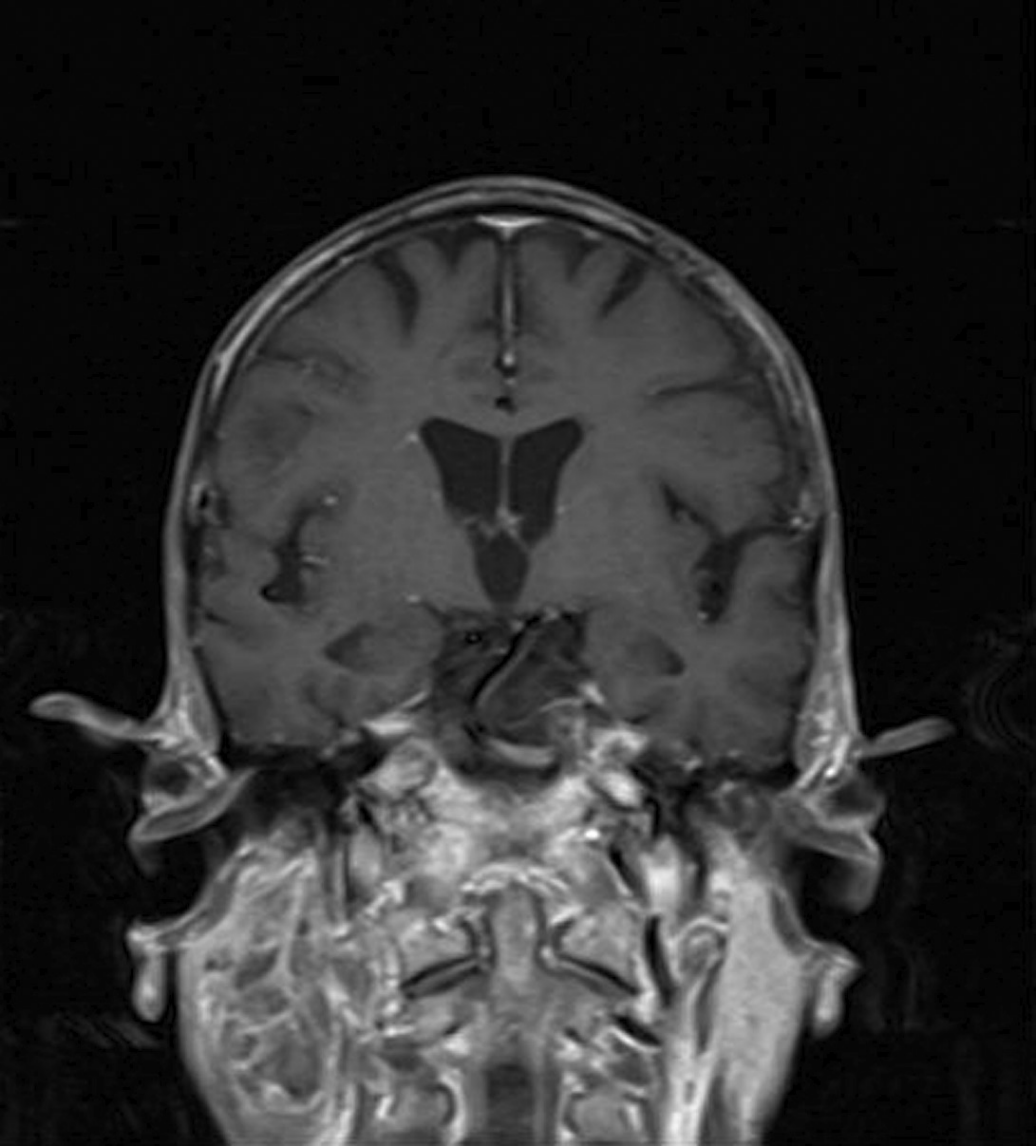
Coronal T1W brain MRI with contrast shows heterogeneous mass legions on the right side, suggesting metastatic LAP
